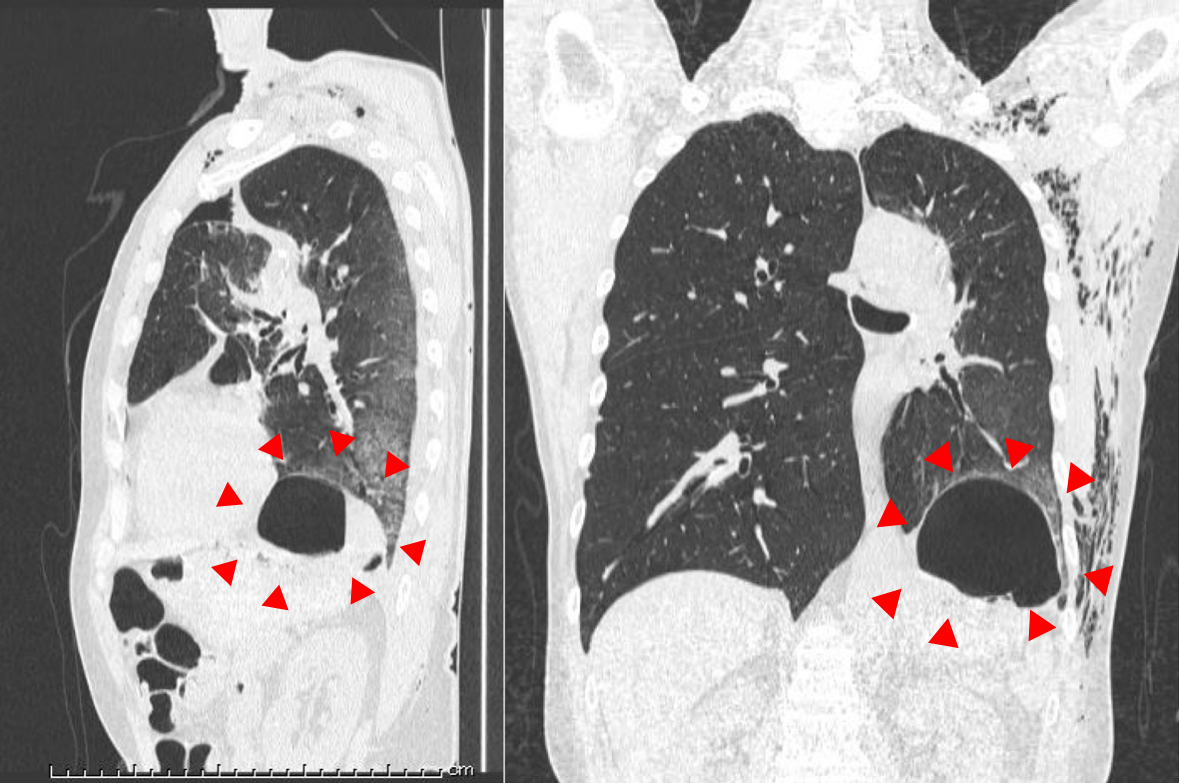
Chest CT reveals a dead space including air and fluid above the left diaphragm (red arrowheads)
